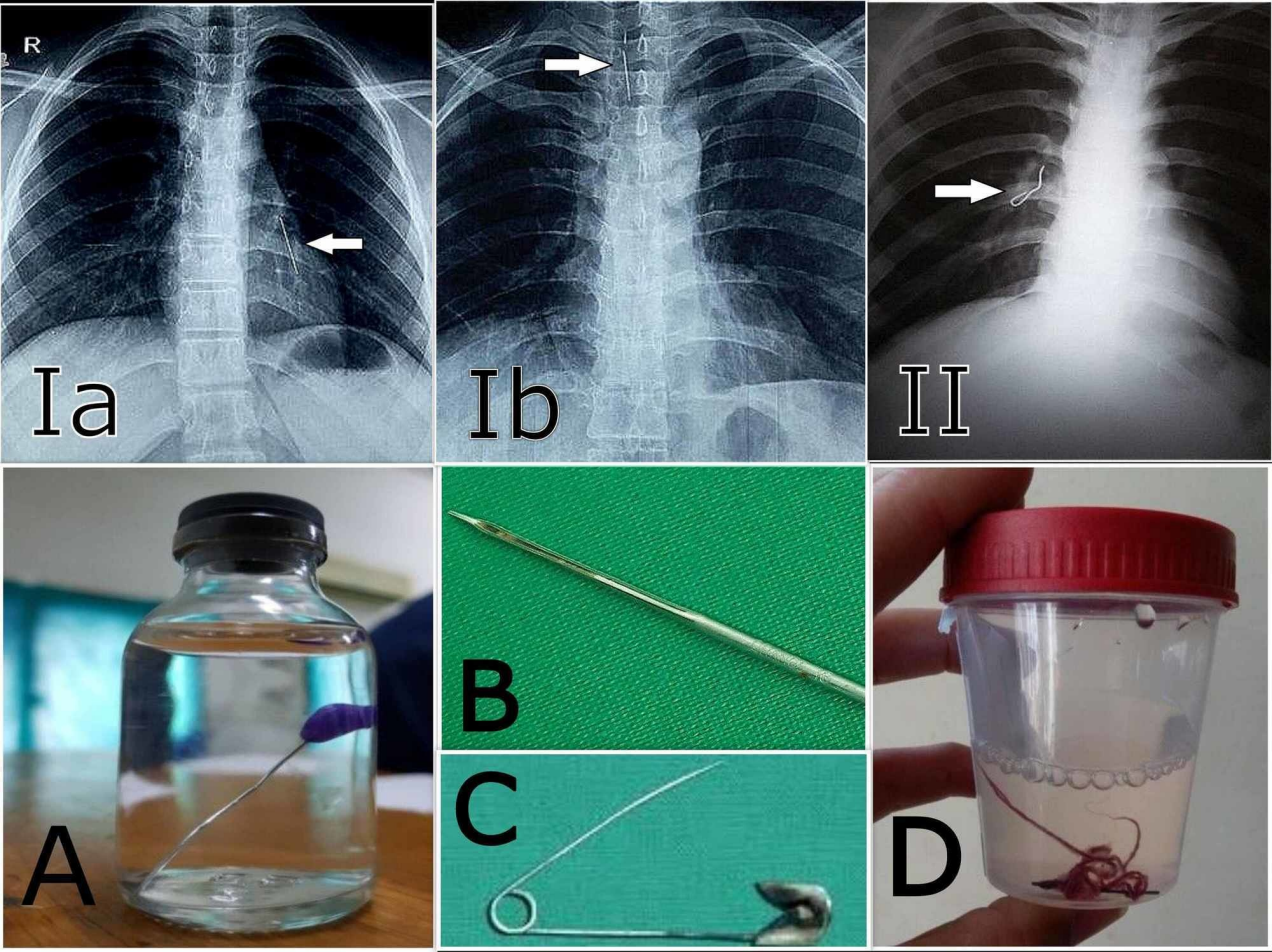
Chest X-rays showing locations of foreign bodies (1a-II); images of different types of foreign bodies removed (A-D) Ia: scarf pin in the left airway; Ib: scarf pin in the trachea; II: sewing needle in the right airway; A: scarf pin; B: tire repair needle; C: safety pin; D: sewing needle
